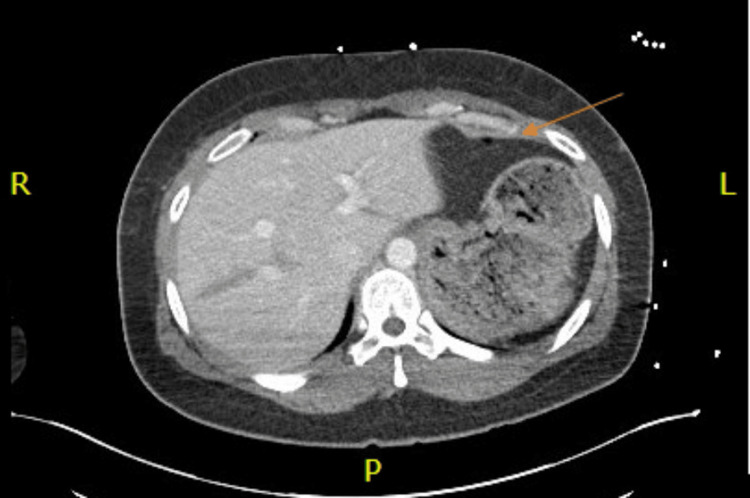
CT axial scan of the chest with intravenous contrast - image 2 The orange arrow shows grade III traumatic liver injury consisting of a laceration in the left lobe extending at least 7 cm with additional parenchymal contusion about the site of laceration without evidence of active bleeding CT: computed tomography
